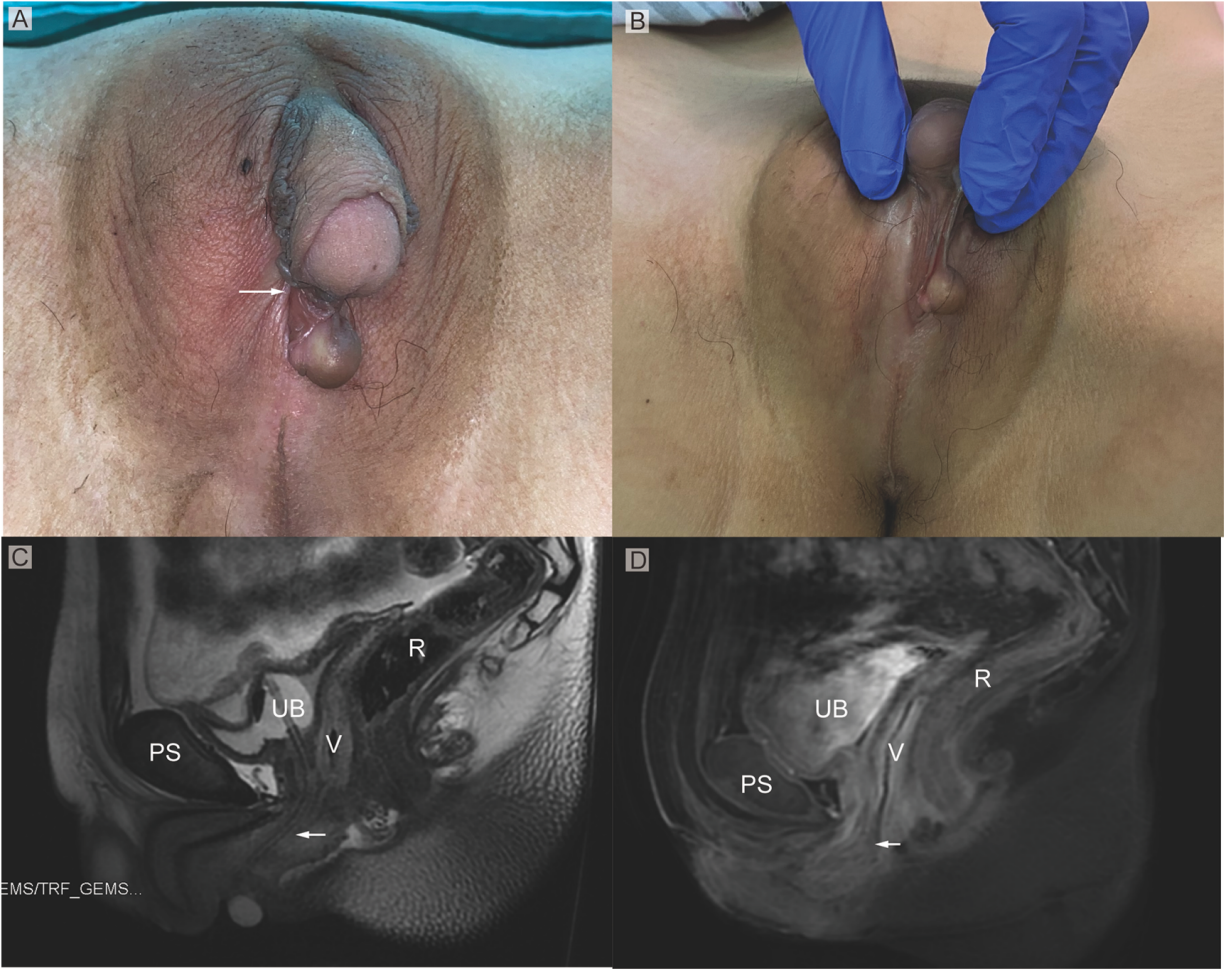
(A) Phenotypic appearance of genitalia before surgery. An enlarged clitoris, completely fused labia majora, and a movable painless mass at the right inguinal region was found. (B) An opening of the urogenital sinus was seen at the base of the clitoris. (C,D) Preoperative contrast-enhanced MRI showing well-defined urogenital sinus. Uterus was not detected. Sagittal MRI scan showing urinary bladder (UB), vagina (V), the common opening of the urogenital sinus (arrow), and rectum (R) The distance between the conference point to the superficial perineal pouch was below the level of the distal end of pubic symphysis (PS).
